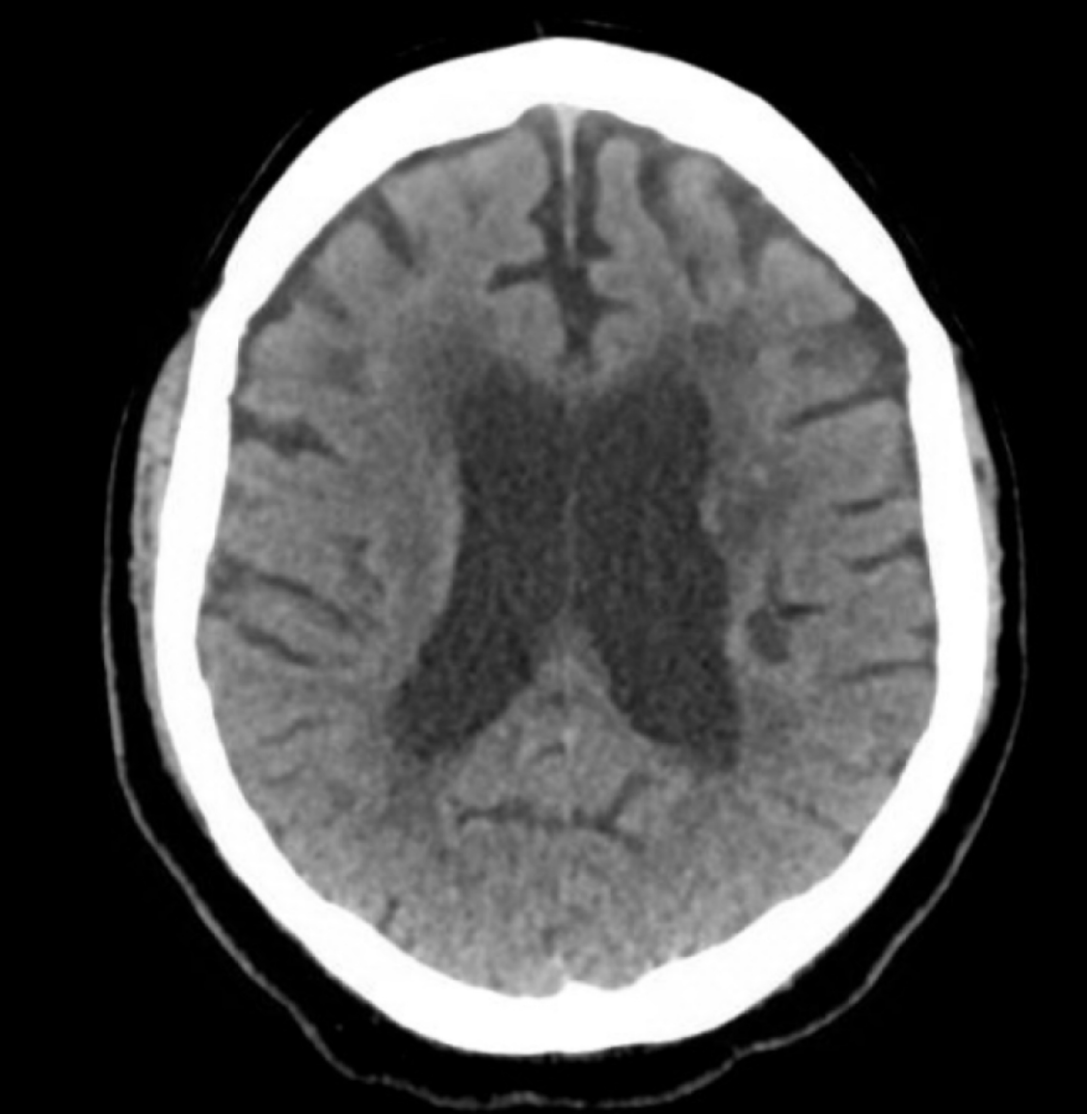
Computed tomography scan brain unremarkable for subarachnoid hemorrhage or any other acute insult
